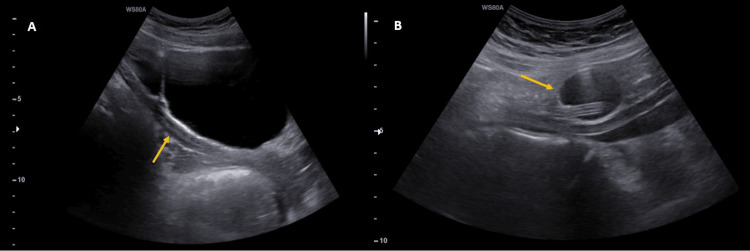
Ultrasonography findings Foley catheter in the ectopic ureter draining to the urethra (A) misplaced balloon of Foley catheter in the right lower quadrant near iliac arteries (B)
